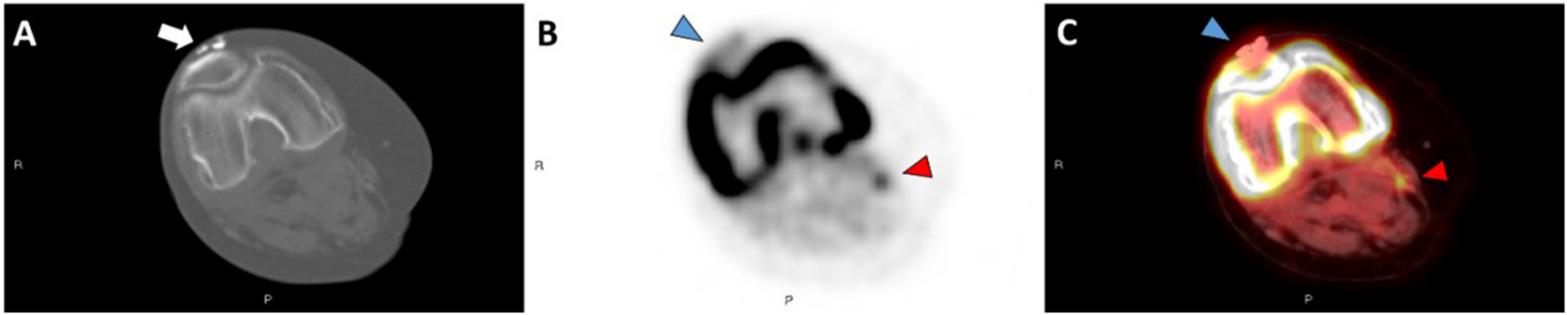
Axial CT image in bone windows (A) demonstrates calcinosis involving the quadriceps and patellar tendon complex overlying the left patella in patient 5. Axial ^18^F-NaF PET/CT images (B,C) demonstrate mild activity along this calcified complex (blue arrowheads). However, there is focal PET signal activity located along the semimembranosus muscle without associated calcification on CT (red arrowheads).
